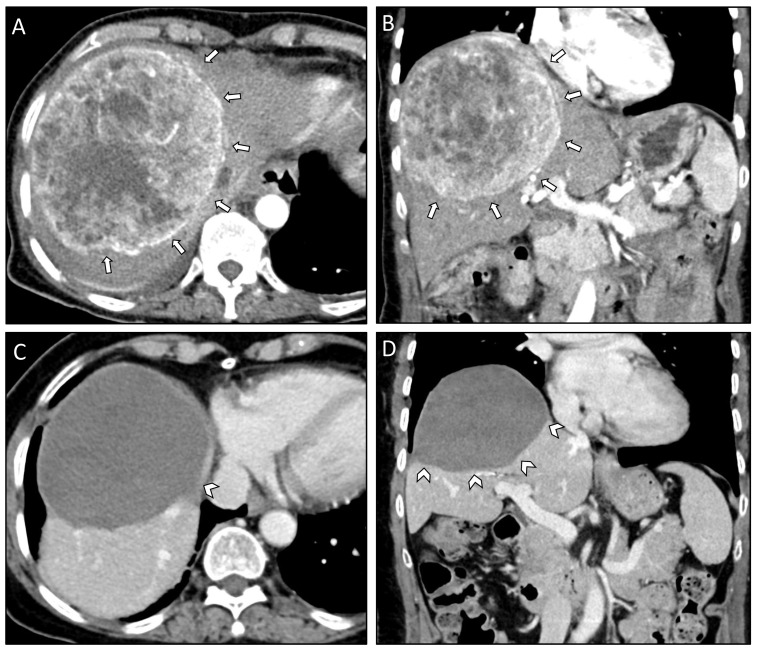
Axial (A) and coronal plane (B) of the pre-interventional CT scan of case #1 depicting a 13 cm measuring SFT/HPC in the right liver lobe (white arrows). Corresponding axial (C) and coronal plane (D) of the last follow-up CT scan 36 months after the initial diagnosis of liver metastasis and 11 months after the last SRFA session with progressively shrinking coagulation zone (white arrowheads).
